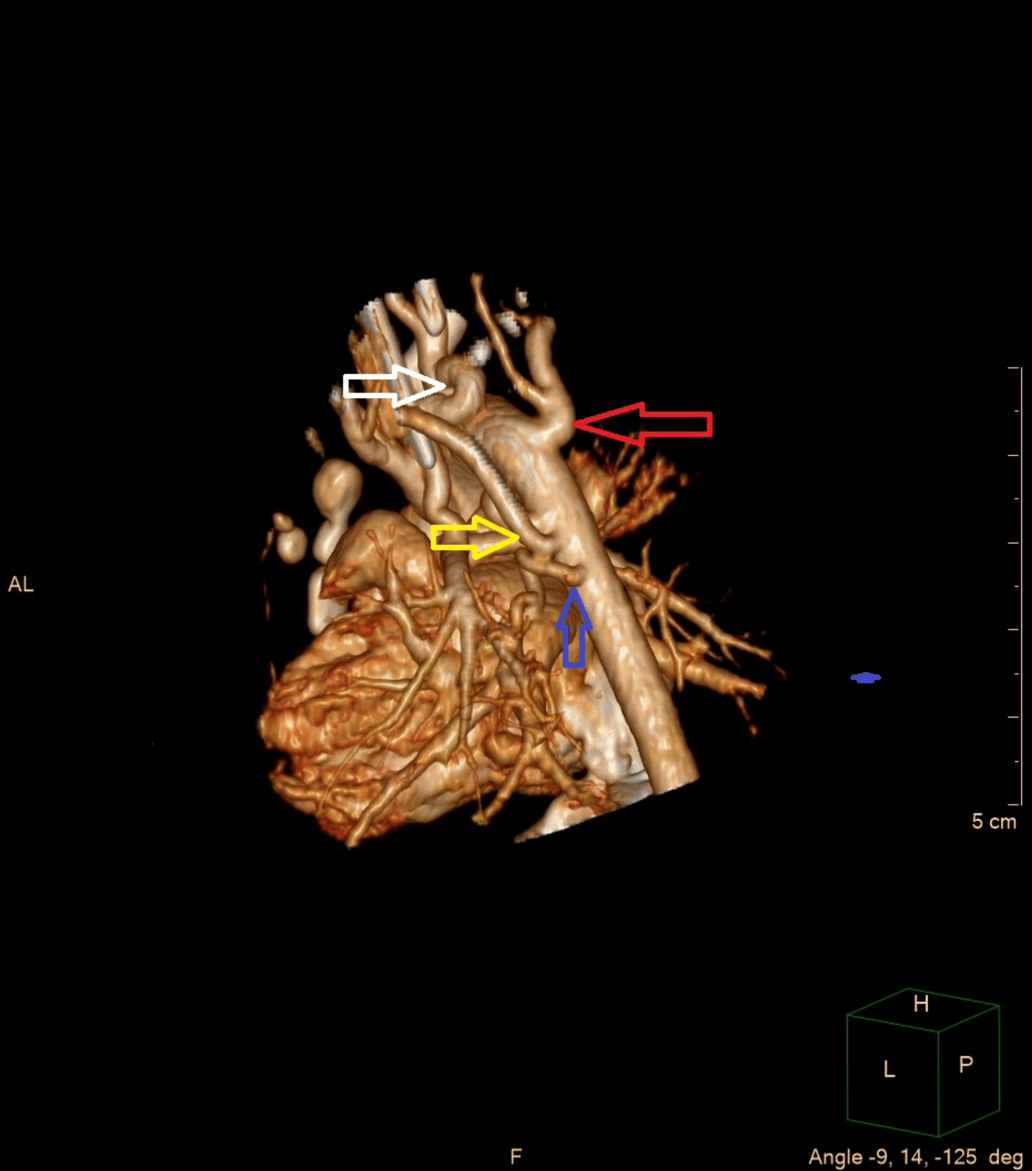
Images from the heart reconstruction created basing on the CT scans (volume-rendered images); back projection The white arrow points to the ductus arteriosus; the red arrow points to the right vertebral artery; the yellow arrow points to the left subclavian artery; the blue arrow points to the one of the MAPCAs. Image credit: Created by the Pediatric Radiology Department team at the University Clinical Center of the Medical University of Warsaw.
